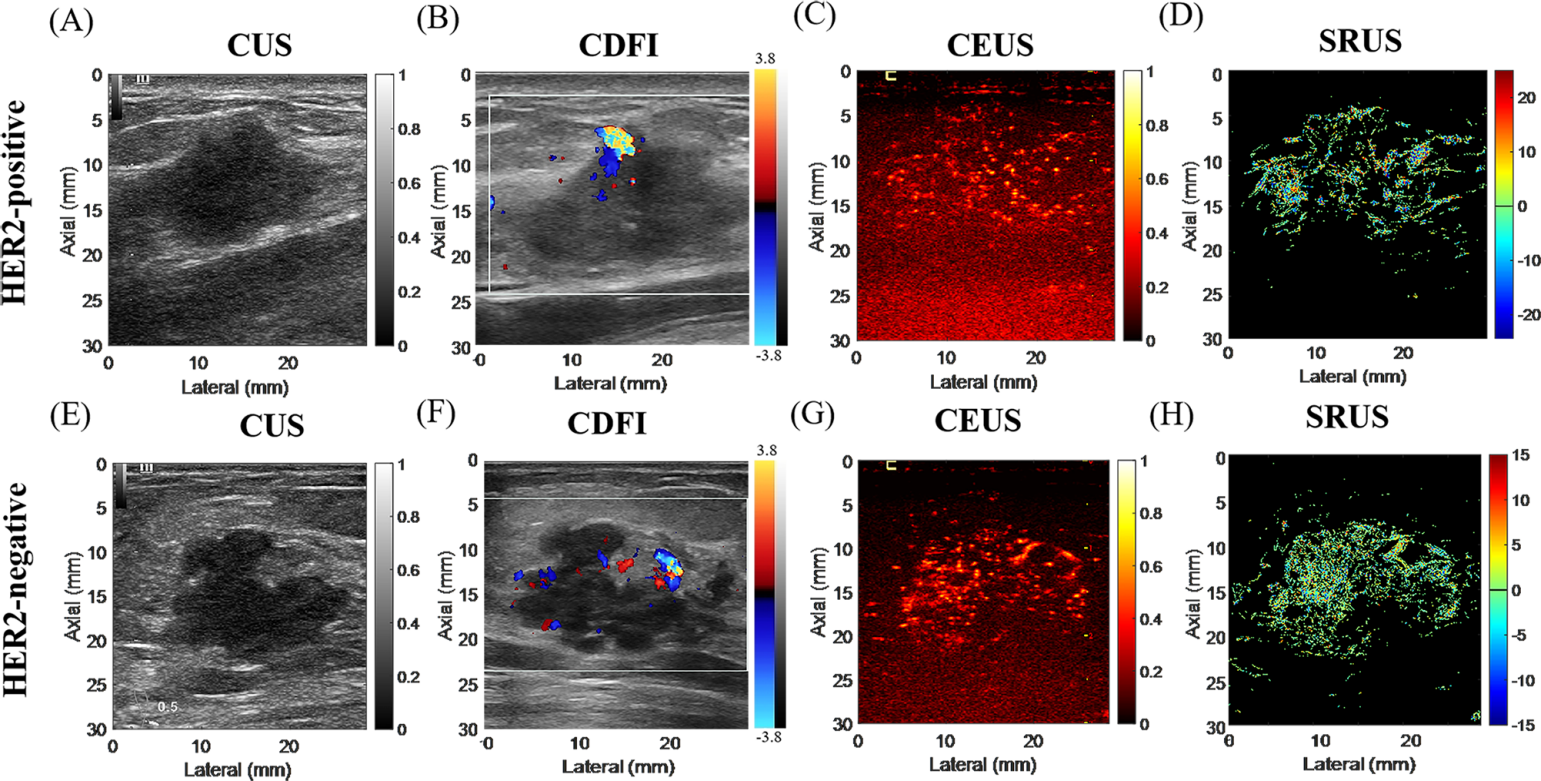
Representative multimodal ultrasound images of breast masses with HER2-positive and HER2- negative. (A–D) correspond to a HER2-positive case: (A) conventional ultrasound image; (B) color Doppler flow image; (C) contrast-enhanced ultrasound image; (D) super-resolution ultrasound image. (E–H) correspond to a HER2-negative case: (E) conventional ultrasound image; (F) color Doppler flow image; (G) contrast-enhanced ultrasound image; (H) super-resolution ultrasound image.
